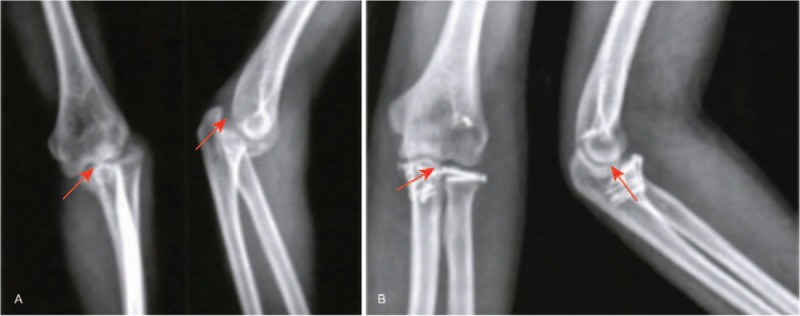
Frontal and lateral X-ray of the elbow joint in a terrible triad of the elbow (TTE) patient caused by a traffic accident before and after surgery, male patient, 42 years old, terrible triad of the right side of the elbow was caused by a traffic accident; the combined lateral and anterior medial approach was used. (A) Frontal and lateral X-ray images before surgery; (B) frontal and lateral X-ray images 6 months after surgery. The red arrow in the figure indicated the injury part.
